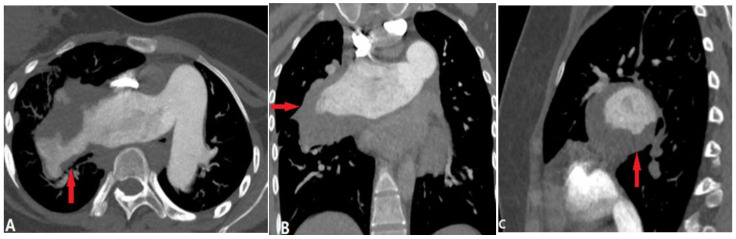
In situ thrombosis in pulmonary hypertension in a 16-year-old girl with primary pulmonary hypertension. (A,B): Axial and coronal CTA images show concentric, broad-based filling defects within the massively dilated right pulmonary artery, and the lumen (blood filling) is narrowed locally (arrow). The main and the left pulmonary arteries are also enlarged. (C): Cross-section of left pulmonary artery in MPR imaging shows interface irregularities (arrow) of the thrombosis within the symmetric widening and smooth outline of the vessel, which rule out compression caused by mass effect. CTA—computed tomography angiography; MPR—multiplanar reformation.
